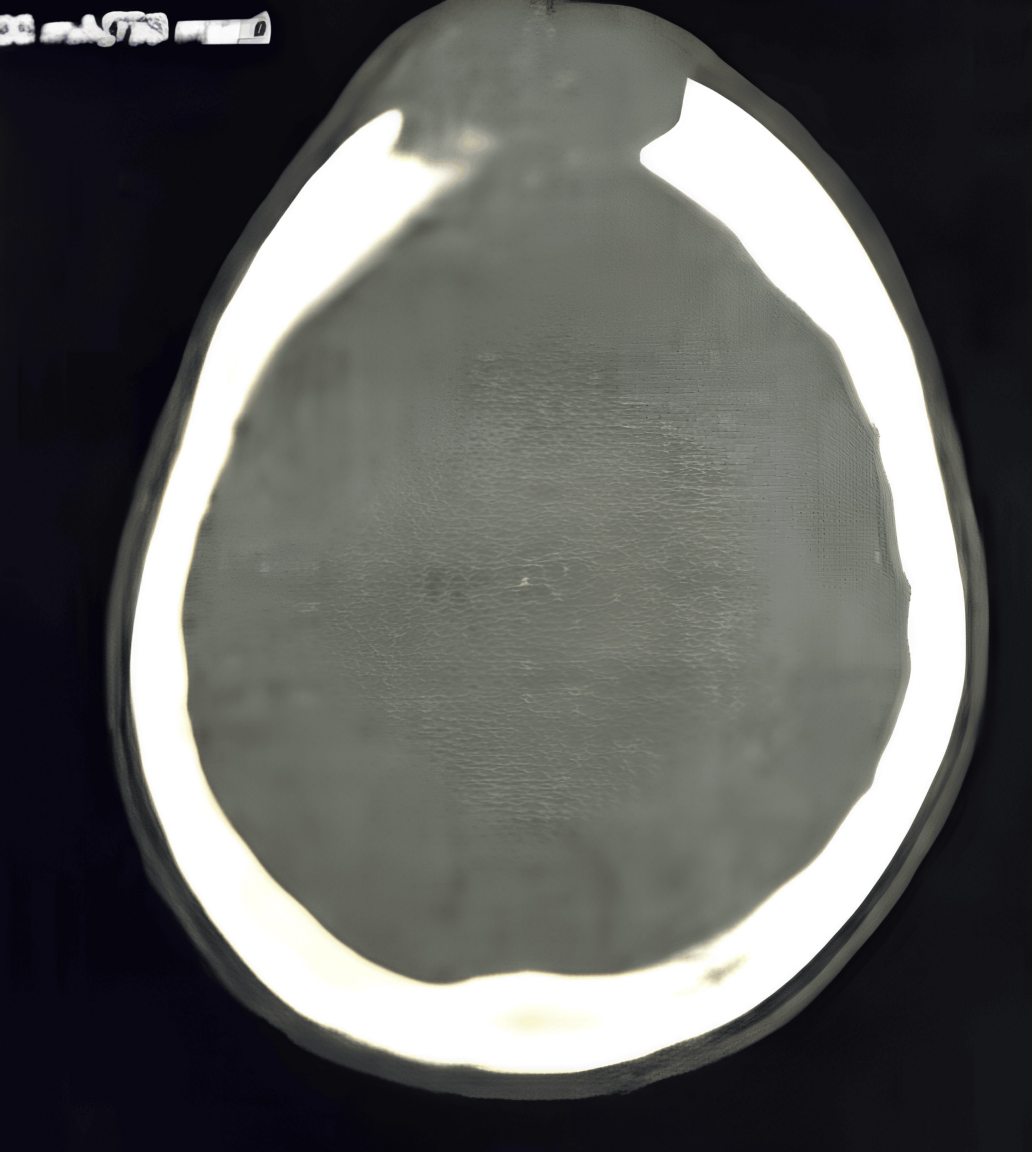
Axial non-contrast brain CT scan showing an osteolytic lesion of the medial frontal osteolytic tissue lesion with the beginning of endocranial extension.
